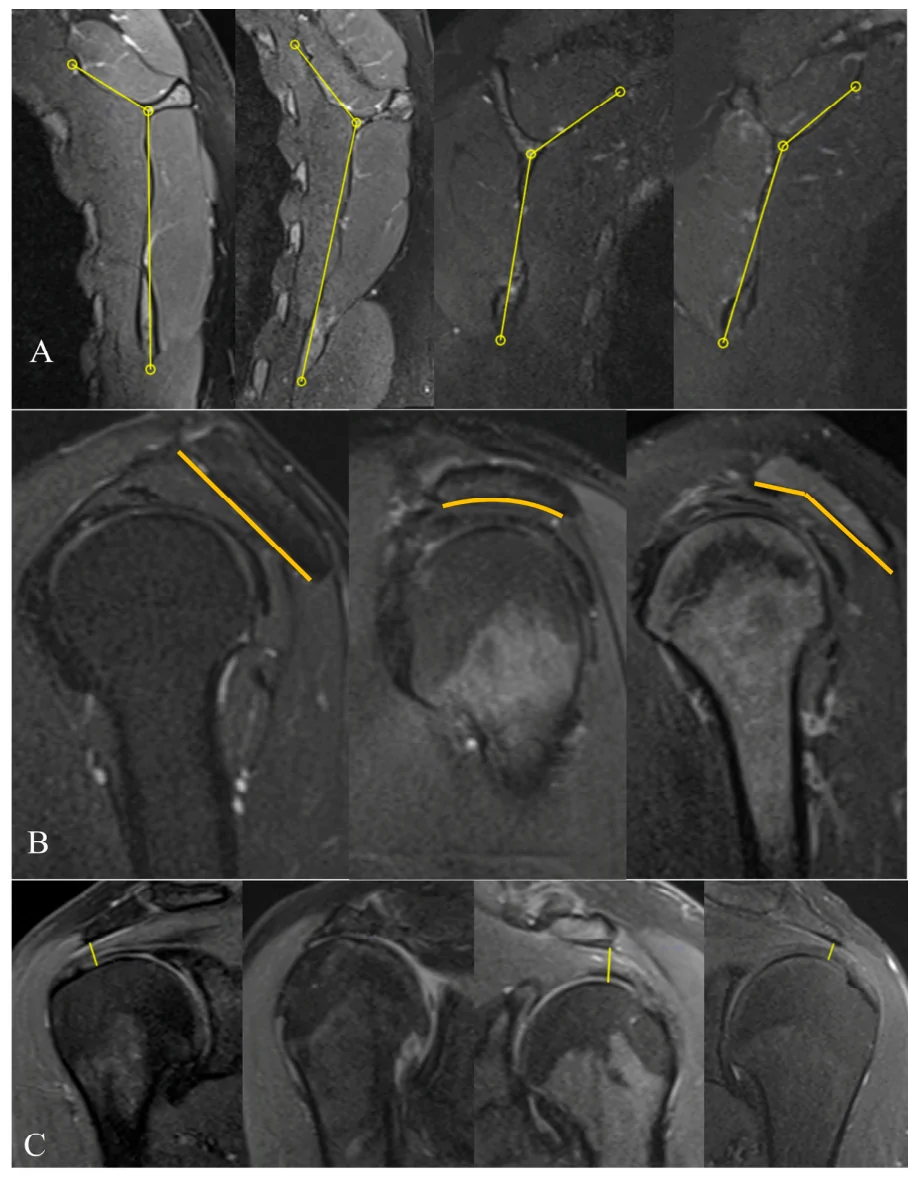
Magnetic resonance imaging (MRI)-based measurement examples: (A) superomedial angle (SMA), (B) acromial morphology, and (C) subacromial space (SAS). The marked lines indicate the SMA measurement in panel (A), the inferior acromial contour in panel (B), and the SAS measurement in panel (C).
